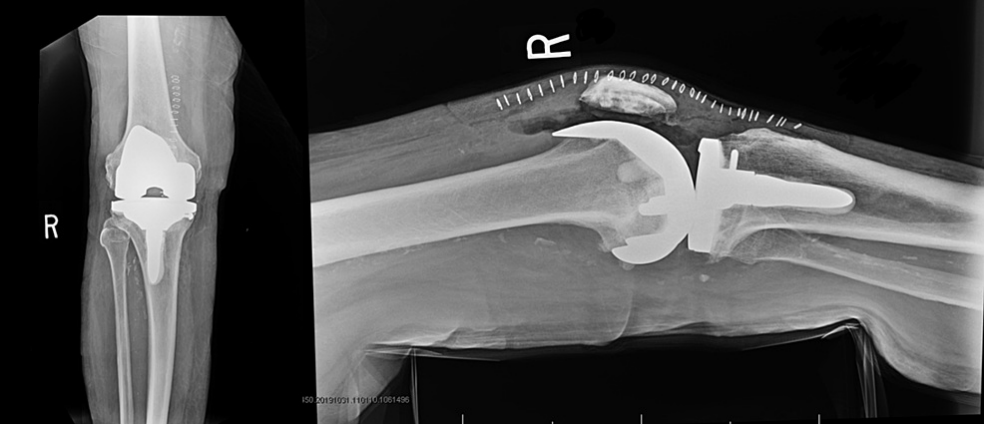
Anteroposterior (Left) and lateral (Right) postoperative radiograph demonstrating a right cruciate retaining TKA with a long stemmed tibial component bypassing the intraoperative tibia fracture. TKA, total knee arthroplasty
